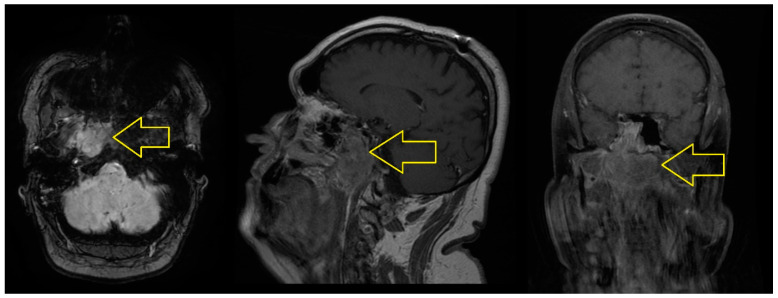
MRI of the cranio-cerebral area with contrast medium in November 2017. The infiltrating tumor mass affects the cavity on the right side, with extension at the base of the cranium (yellow arrow).
